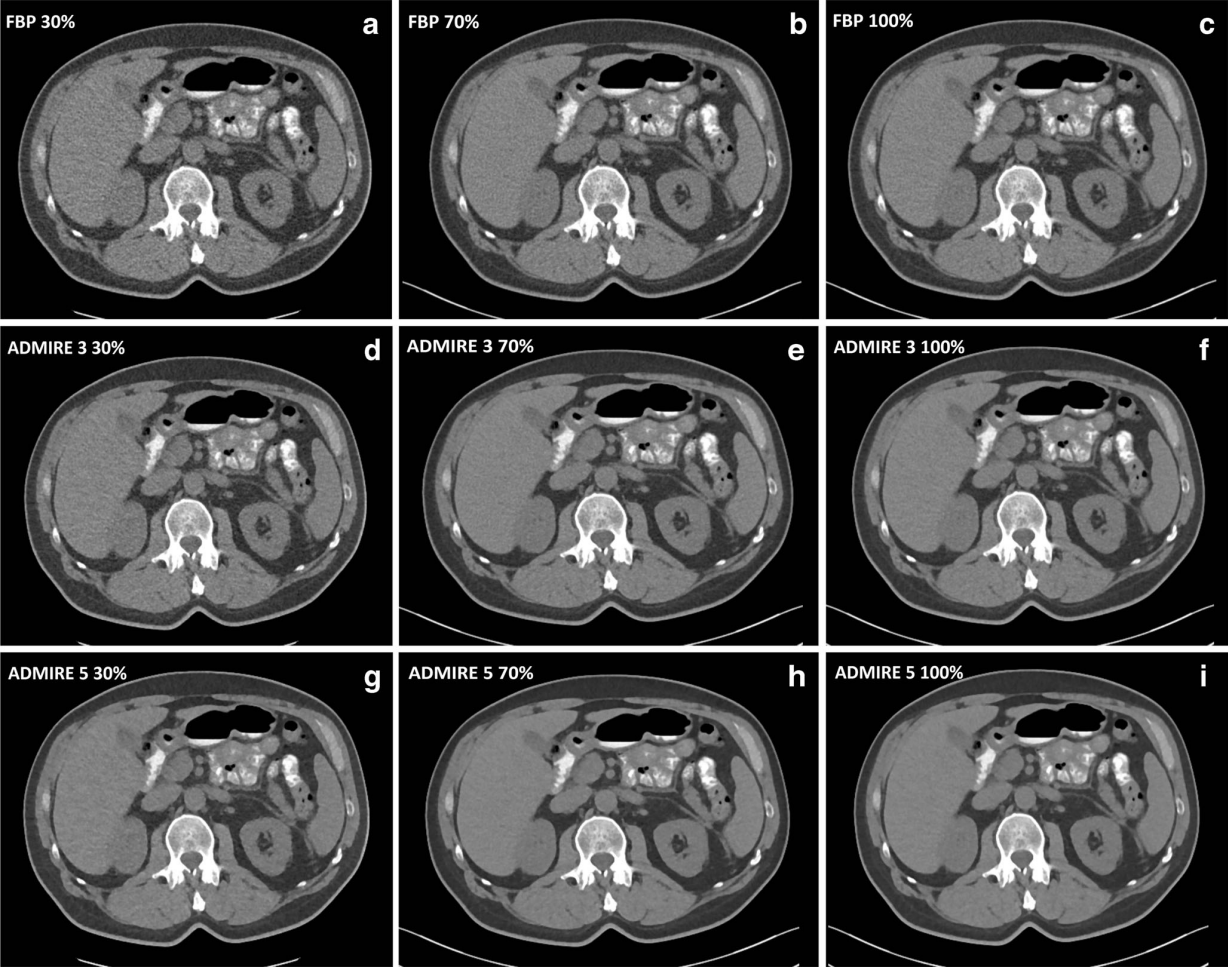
Transverse CT sections at 30, 70 and 100% tube loads reconstructed with (a) filtered back projection (FBP) 30%, (b) FBP 70%, (c) FBP 100%, (d) ADMIRE 3 30%, (e) ADMIRE 3 70%, (f) ADMIRE 3 100%, (g) ADMIRE 5 30%, (h) ADMIRE 5 70% and (i) ADMIRE 5 100% in a 59-year-old male patient with a body mass index (BMI) of 24.8 kg/m^2^
